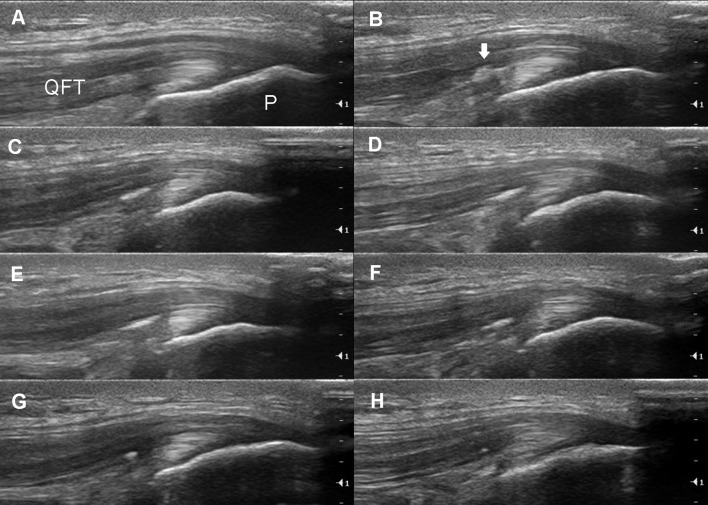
Longitudinal ultrasound imaging of the same subject. (A) The normal right quadriceps femoris tendon (QFT) and its insertion into the patella (P). (B) Intratendinous collections of bright foci (arrow) can already be clearly visualized after two jumps. Dynamic changes in the intratendinous foci can be seen after 15 (C), 40 (D), 75 (E), and 100 (F) jumps and 20 min (G) and 40 min (H) after exercise.
